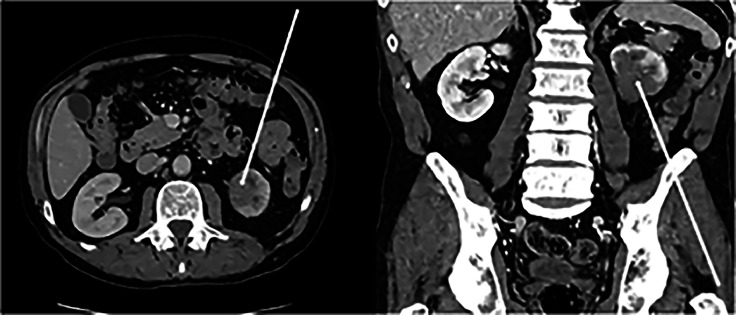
Panel 2a (axial CT image in portal venous contrast phase) demonstrating an endophytic hilar hypodense heterogenous mass measuring 33mm (as indicated by white arrow). Panel 2b (coronal CT in portal venous contrast phase) demonstrating the proximity of the mass extending from the lower pole into to the renal hilum with obliteration of typical hilar anatomy (as indicated by white arrow).
